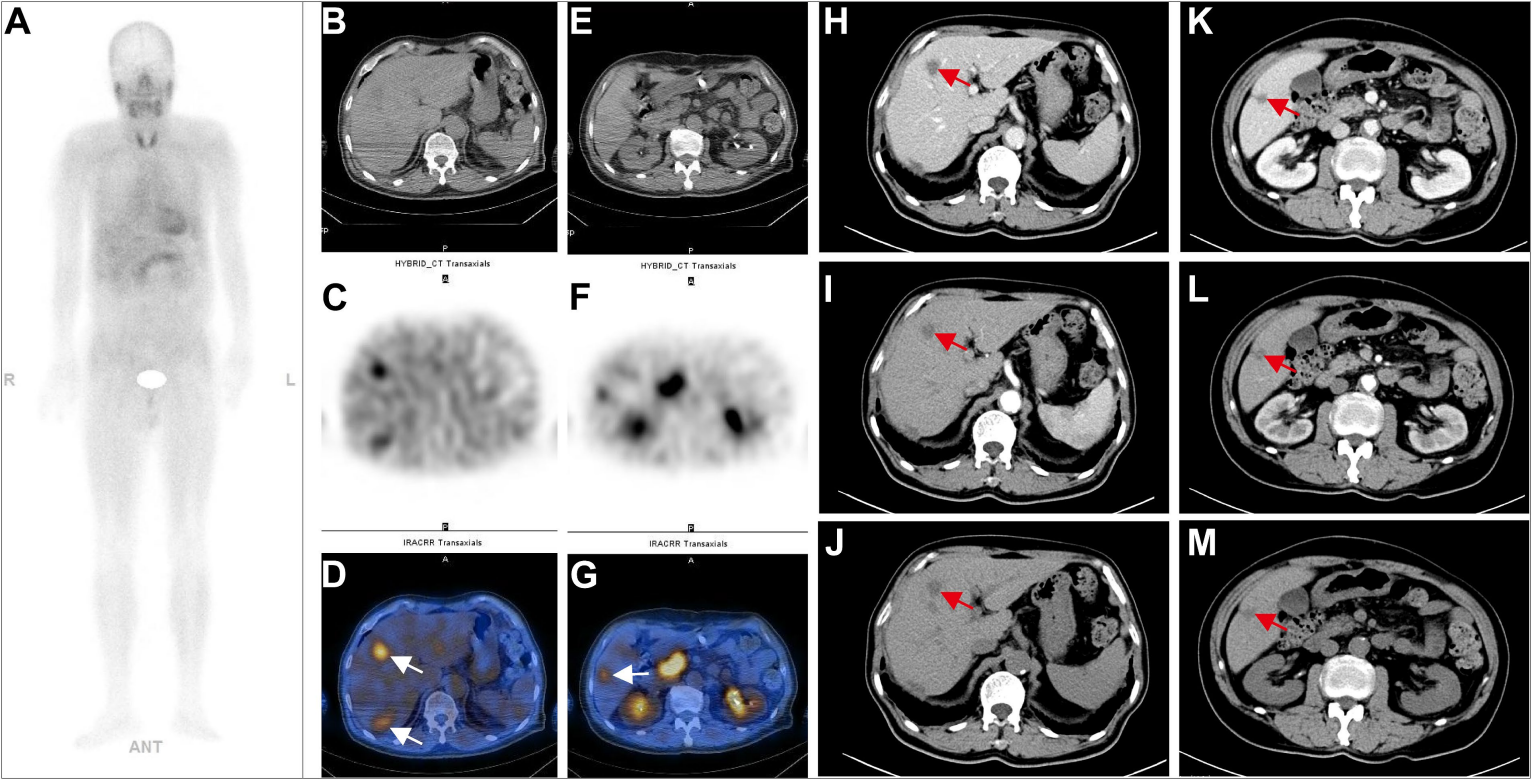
A 73-year-old postoperative male patient with sigmoid colon adenocarcinoma (PT3N1M1, stage II) underwent ^99m^Tc-FAPI-04 SPECT/CT and CECT examinations. The whole-body MIP image (A). The axial CT image (B,E), axial SPECT image (C,F), and the corresponding fused ^99m^Tc-FAPI-04 SPECT/CT image (D,G). In the liver, multiple low-density lesions with unclear margins and high uptake of ^99m^Tc-FAPI-04 were observed on whole-body SPECT/CT. In abdominal CECT, multiple slightly low-density nodular shadows with unclear margins, which exhibited uneven enhancement but clear margins after contrast, can be seen in the left lobe (H–J) and the right posterior segment (K–M) of the liver, consistent with the imaging manifestations of liver metastases. The white arrow indicates ^99m^Tc-FAPI-04 uptake in the lesion, and the red arrow indicates the metastatic foci in the liver.
